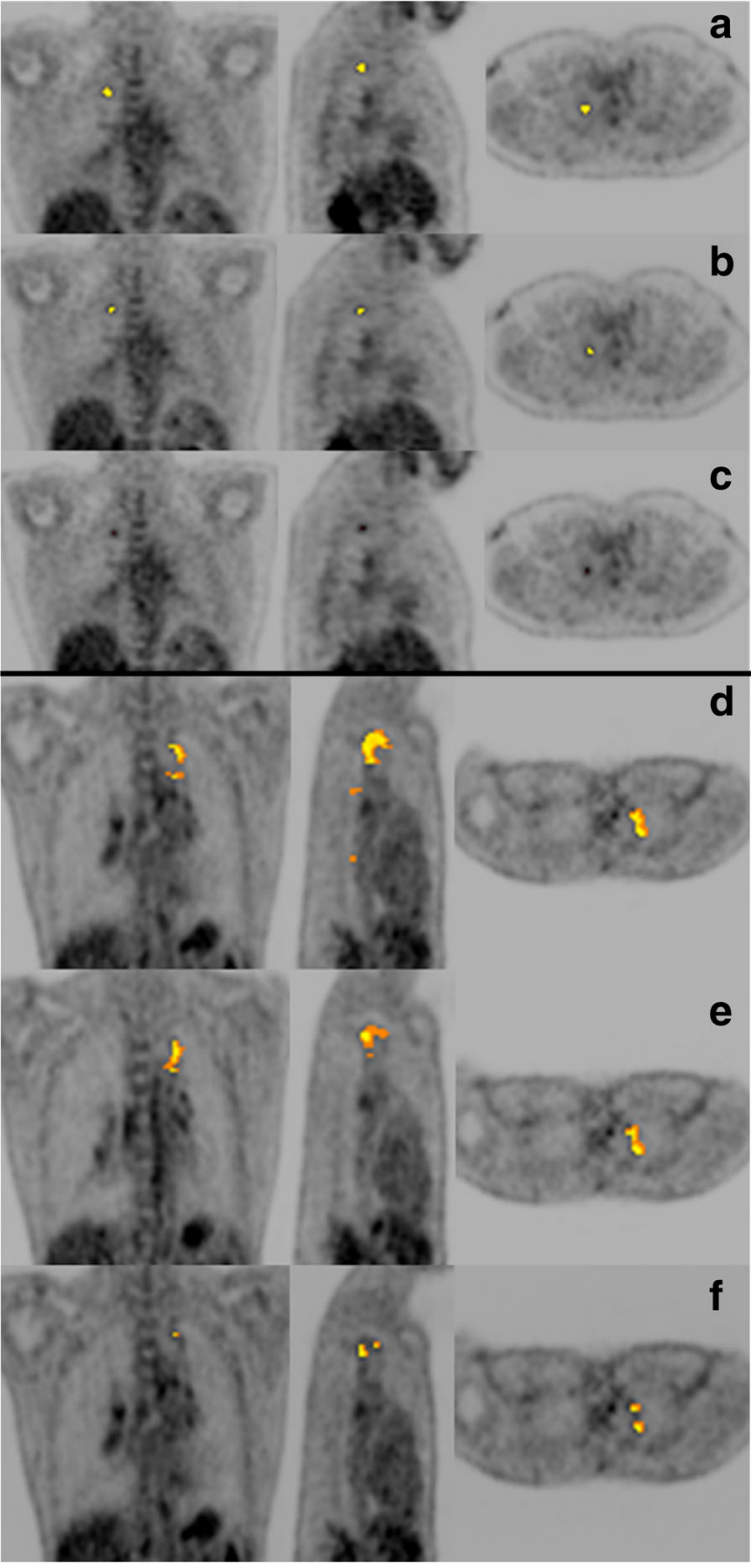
Coronal (left), sagittal (middle) and transverse views of PET scans of two patients after treatment for pulmonary tuberculosis. The first patient (a–c) has a residual nodule in the right upper lobe showing minimally increased FDG avidity. The second patient (d–f) has a complex lesion in the left upper lobe with mild FDG avidity. The auto-delineated metabolic lesion volume is overlaid using ascending *Z*-score thresholds, respectively at *Z* = 7 (a, d), *Z* = 8 (b, e) and *Z* = 9 (c, f)
